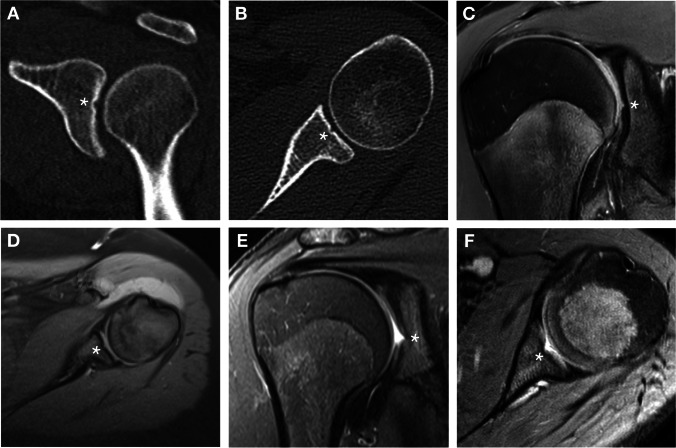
The appearance of the osseous depression (asterisk) on CT and MRI scans. CTs showing coronal and axial slices of a 25-year-old female (A, B). MRIs displaying the osseous depression covered with articular cartilage in coronal and axial slices of a 17-year-old male (C) and a 16-year-old female (D), respectively. MRIs showing a fluid-filled osseous depression in coronal and axial slices of two 15-year-old males (E, F)
